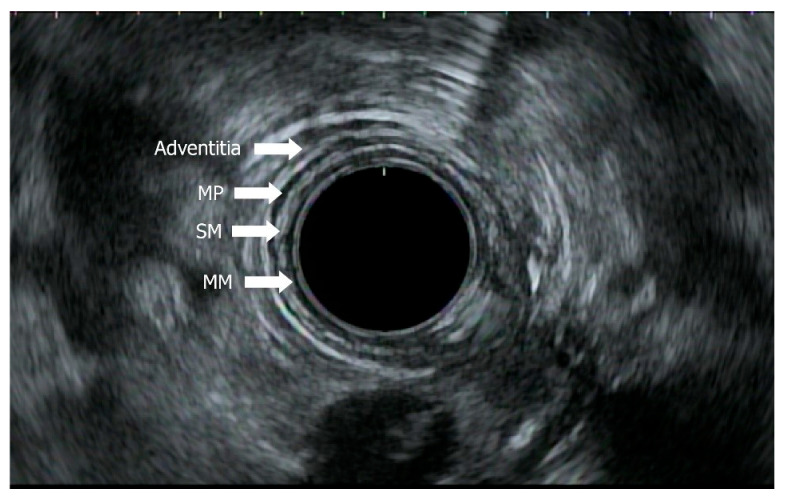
Endoscopic ultrasound of normal esophageal wall layers. MM: mucosa; SM: submucosa; MP: muscularis propria.
